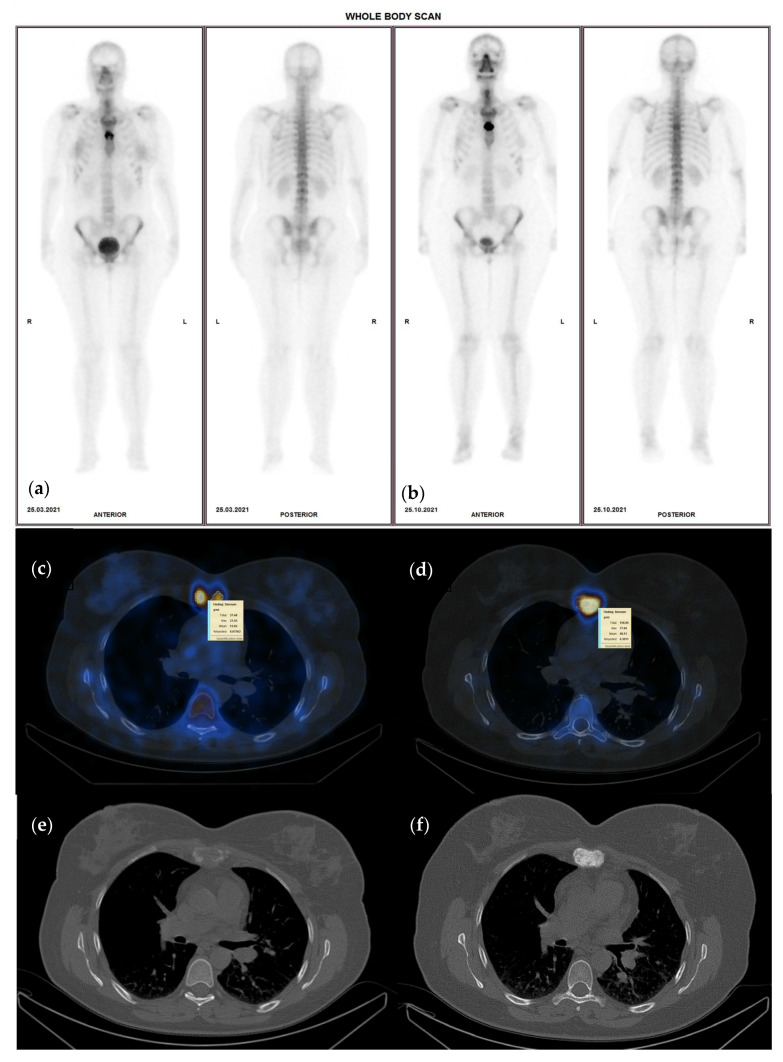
Sixty-nine-year-old female with invasive ductal carcinoma and surgical (right mastectomy), chemotherapy and hormone therapy treatment presenting with bone metastases, represented by high heterogenous radiotracer uptake on the sternum with an SUVmax of 23.5 g/mL (a)—WBS and (c)—hybrid image corresponding to an osteolytic lesion on CT—(e), on the baseline study; (b)—WBS, (d)—hybrid image of the follow-up SPECT-CT after 8 months of osteoclast inhibitor treatment, presenting higher radiotracer uptake (SUVmax of 77.8 g/mL) although the lesion became osteoblastic on the CT scan (f).
